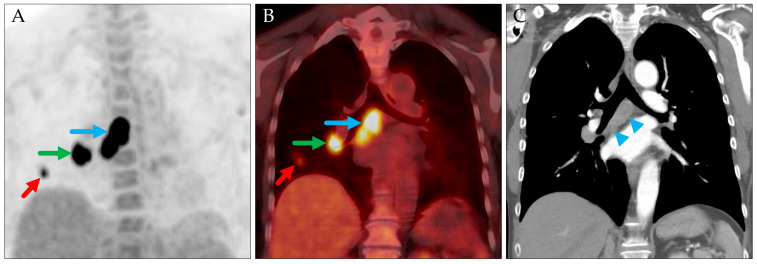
PET maximum intensity projection (MIP) in anterior–posterior (AP) view (A), coronal PET/CT (PET fused with attenuation-correction CT) (B), and coronal contrast-enhanced CT (C) acquired in the same session. The primary tumor is in the right lower lobe (red arrows; cT1b), right hilar (green arrows), and right subcarinal (blue arrows) lymph nodes (cN2). Contrast-enhanced CT confirms multiple nodes (blue arrowheads), but all are located within a single station (station 7) and are therefore are classified as cN2a according to TNM-9.
